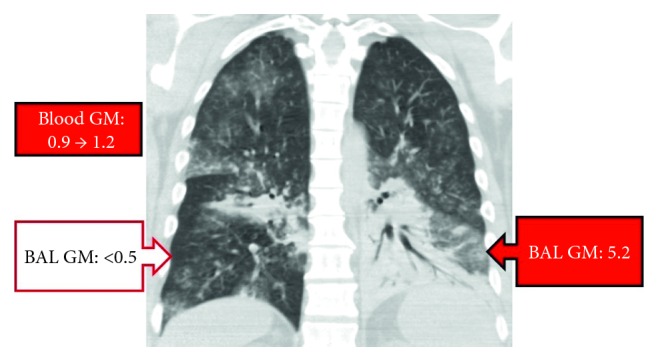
CT scan of the chest. Multifocal dense lung consolidations, most prominent in the left lower lobe, tree in bud opacities, and centrilobular nodules in the right lung; serum and left lower lobe: BAL galactomannan (GM) positive; right lower lobe: GM negative.
